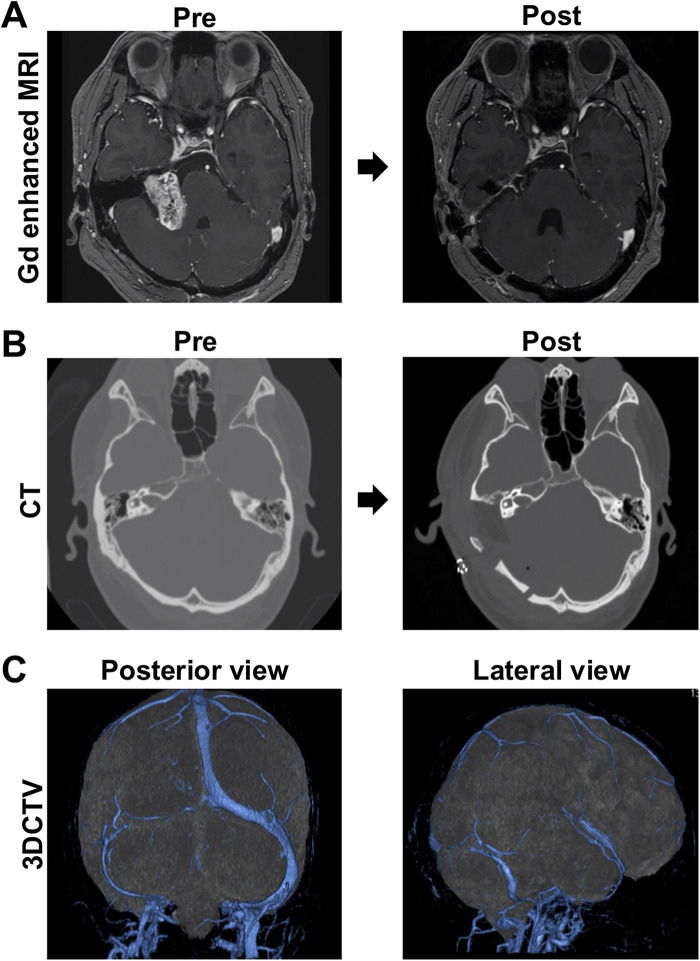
Preoperative and postoperative radiographic findings. MRI demonstrates a 40 mm × 27 mm × 29 mm tumor showing heterogeneous enhancement, extending vertically toward the brainstem and cerebellar peduncle along the surface of the petrous bone, with significant compression of the brainstem. Gross total resection was maintained without evidence of recurrence on MRI at approximately 6 months postoperatively (A). Postoperatively, the internal auditory canal was opened, and mastoidectomy was performed up to the area immediately adjacent to the semicircular canals (B). Preoperative three-dimensional CT venography, viewed from an oblique posterior angle, demonstrates that the ipsilateral sigmoid sinus is present but smaller in caliber than the contralateral side (C).
